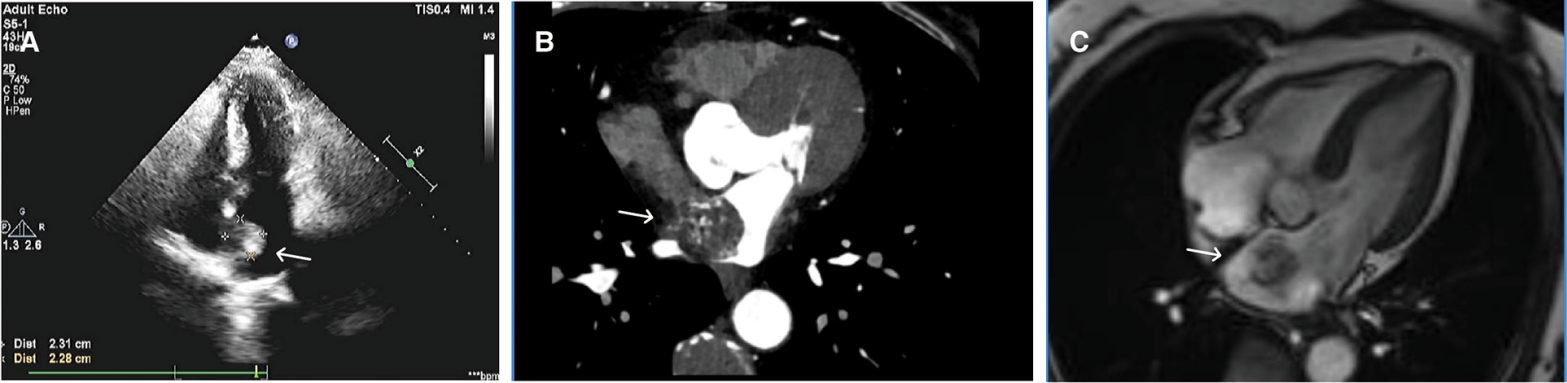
Preoperative echocardiography, coronary CTA, and cardiac MRI. (A) Echocardiography showed a medium echogenic mass attached to the upper part of the interatrial septum in the left atrium, with a wide base, regular shape, and fixed position. (B) Coronary CTA demonstrated a spherical lesion in the left atrium with right coronary artery blood supply. (C) LGE imaging depicted a spherical mass having a size of approximately 2.5 cm × 2.6 cm × 2.7 cm in the left atrium, with an abnormal signal showing a clear edge and patchy and uneven delayed enhancement.
